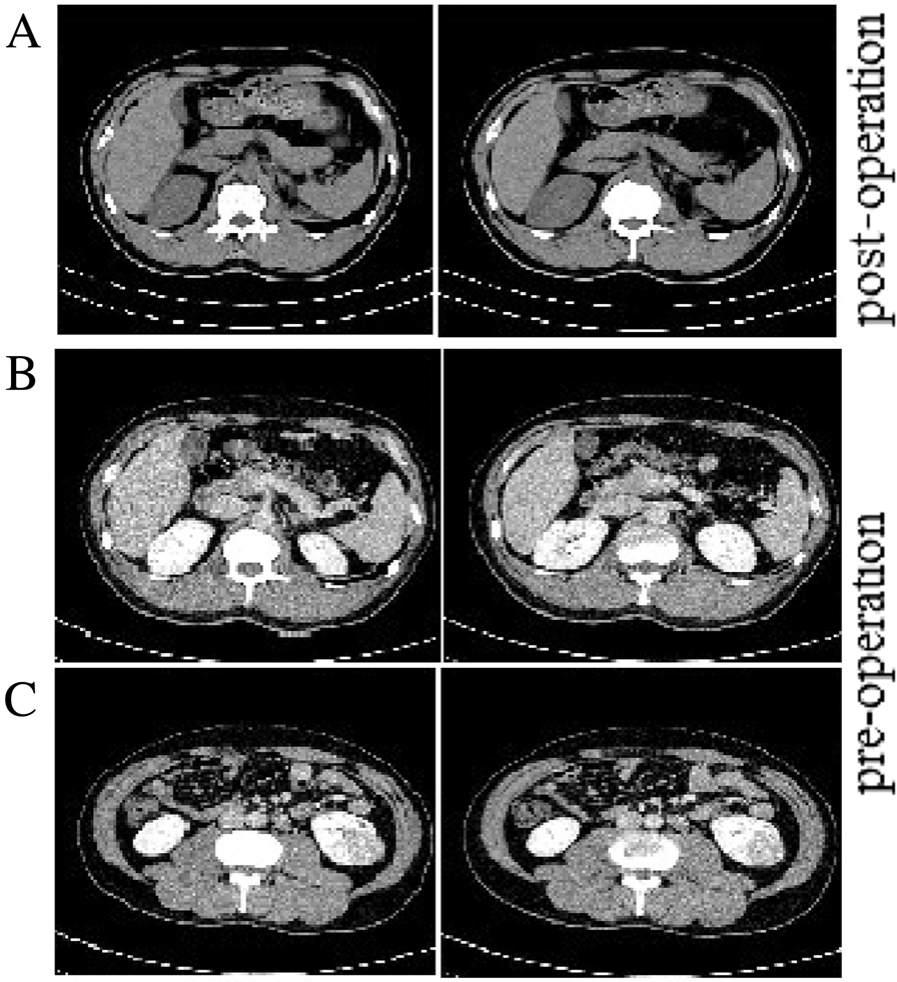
Computed Tomography (CT) examination of a 43-year-old male with left renal carcinoma. A. CT imaging at the postoperative check indicated the size, location and integrality of the left adrenal gland. B. CT imaging at the preoperative examination showed the relationship between the left kidney and the left adrenal gland. C. The preoperative CT imaging displayed the location and size of the tumor.
